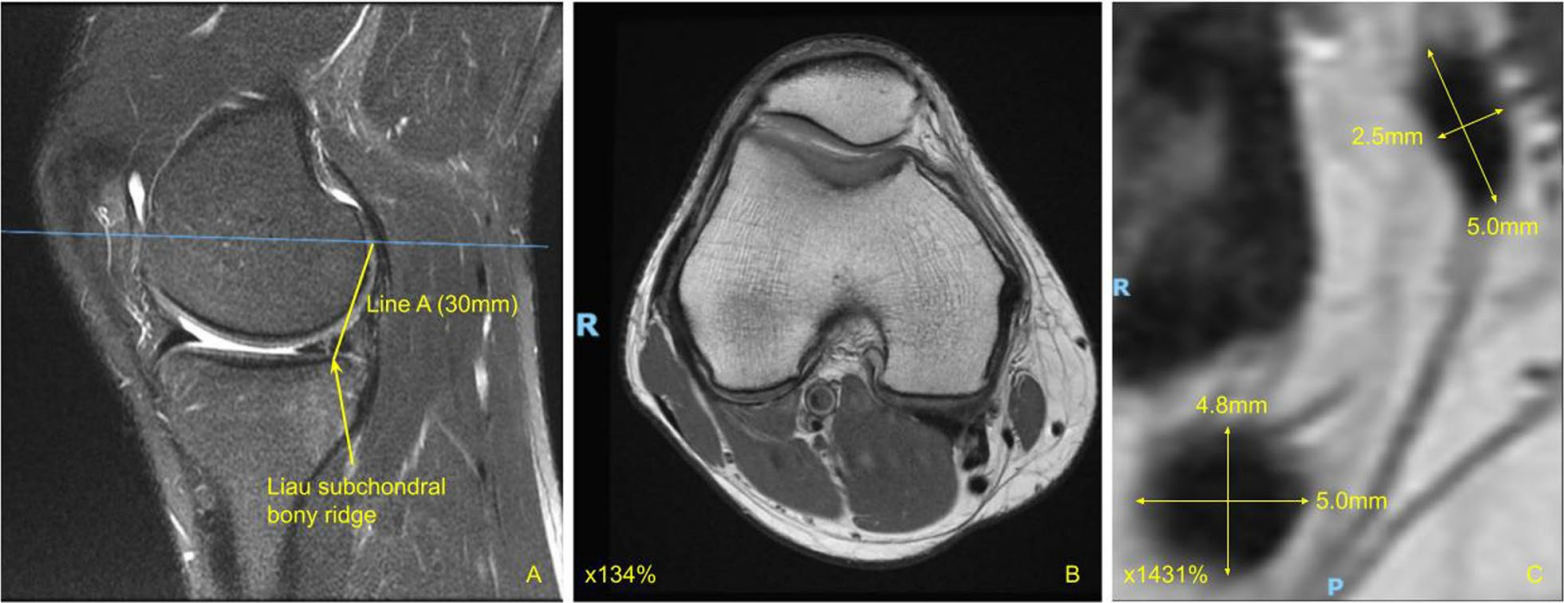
T2-weighted MRI showing sagittal and axial cuts of the affected knee
